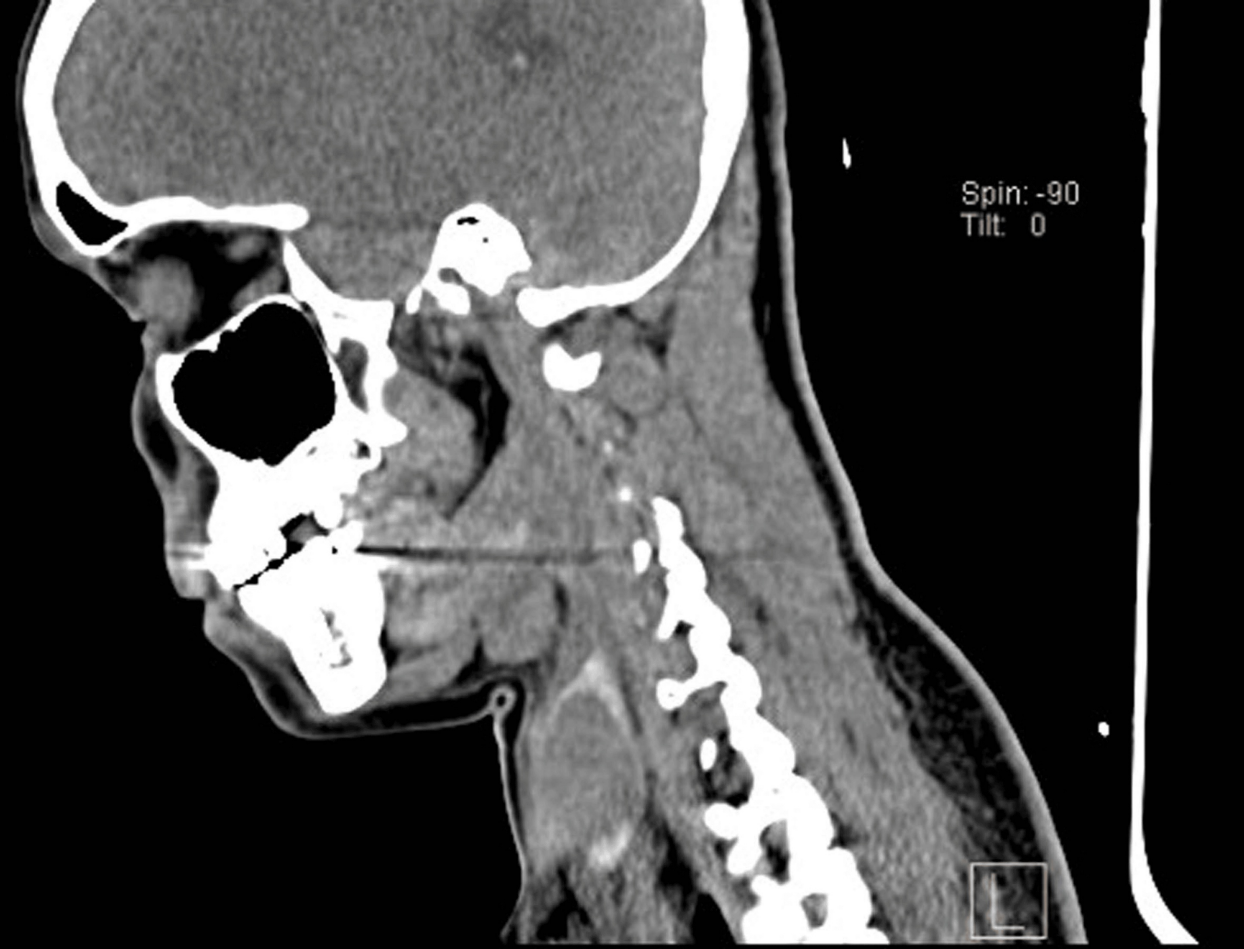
Computed tomography shows a solid cystic lesion within the right lobe of the thyroid.
